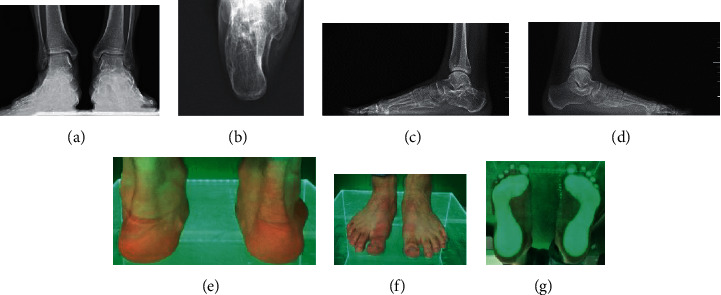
(a–g) Results of imaging tests (X-ray) and podoscopic tests: patient no. 7. (a) Anterior-posterior X-ray, numerous overload changes in the upper ankle joints, tarsometatarsal joints, and calcifications. (b) Axial X-ray: heel bone fracture. (c) Lateral X-ray: numerous overload changes and calcifications. (d) Lateral X-ray: numerous overload changes and calcifications. (e) Posterior photo: ankle joint and hindfoot evaluation. (f) Anterior picture of the feet: assessment of toe positioning and deformation. (g) Plantar photo on the podoscope: assessment of plantar part of the foot and height measurement of the longitudinal arch.
